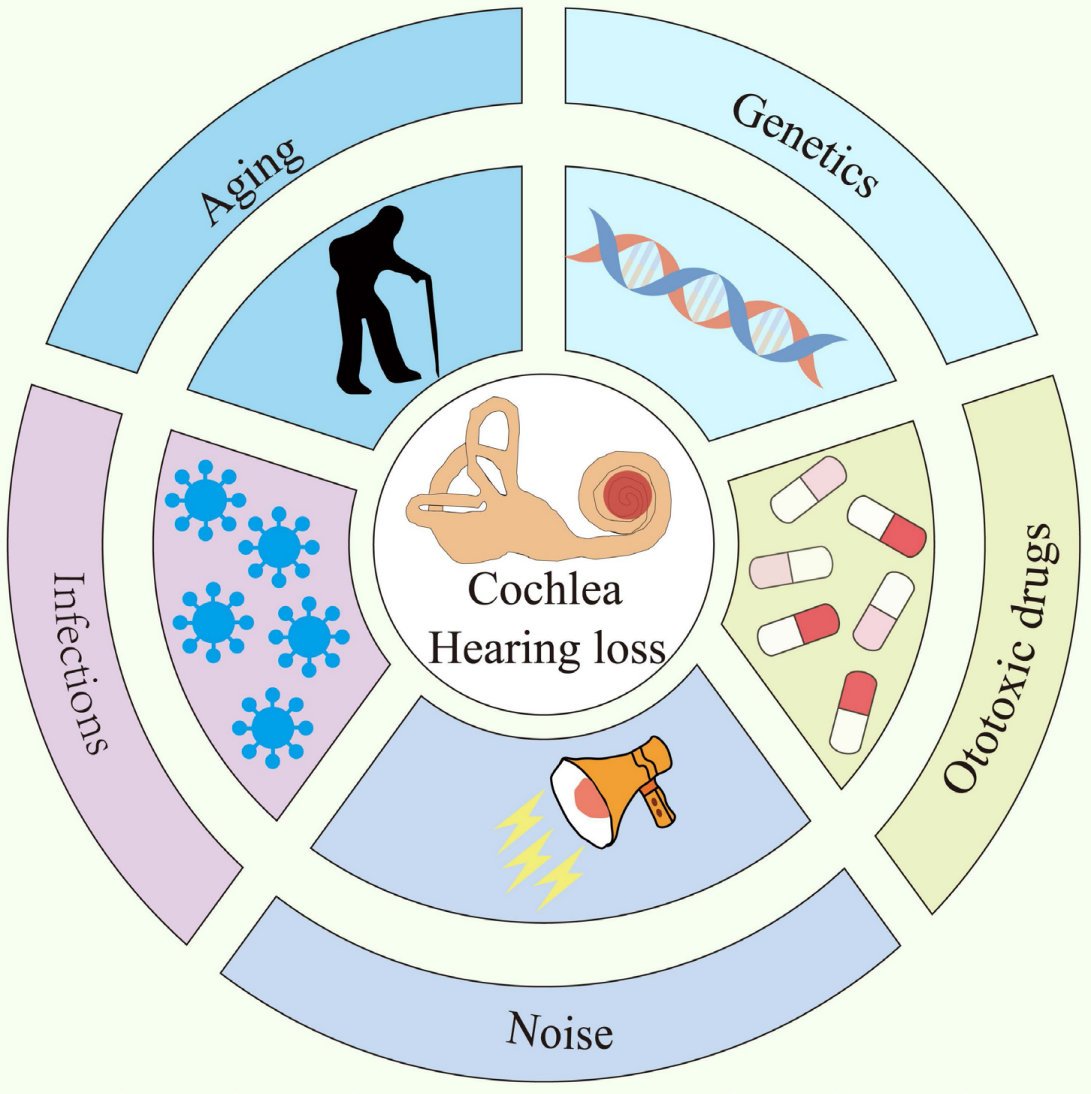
Comprehensive therapeutic strategies and prospects targeting the auditory nerve: focusing on neuroprotection, regulation of the regenerative microenvironment, delivery of neurotrophic factors, synergistic repair via gene editing/stem cell transplantation, and innovations in artificial auditory reconstruction technologies. These strategies aim to surpass the limitations of traditional cochlear implants and envision the clinical translation of emerging technologies such as optogenetics, ultrasound, flexible electrodes, and organoid-based approaches.
